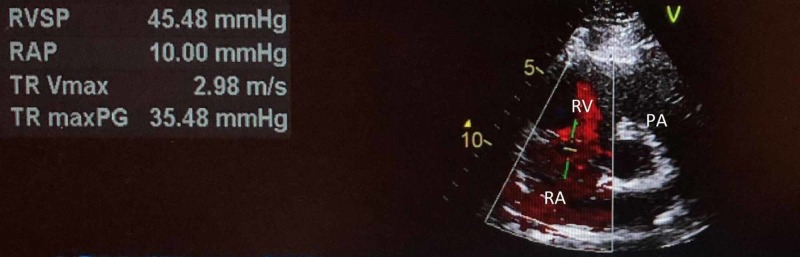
Echocardiogram Right Atrium (RA), Right Ventricle (RV), Pulmonary Artery (PA), Right Ventricle Systolic Pressure (RVSP)
